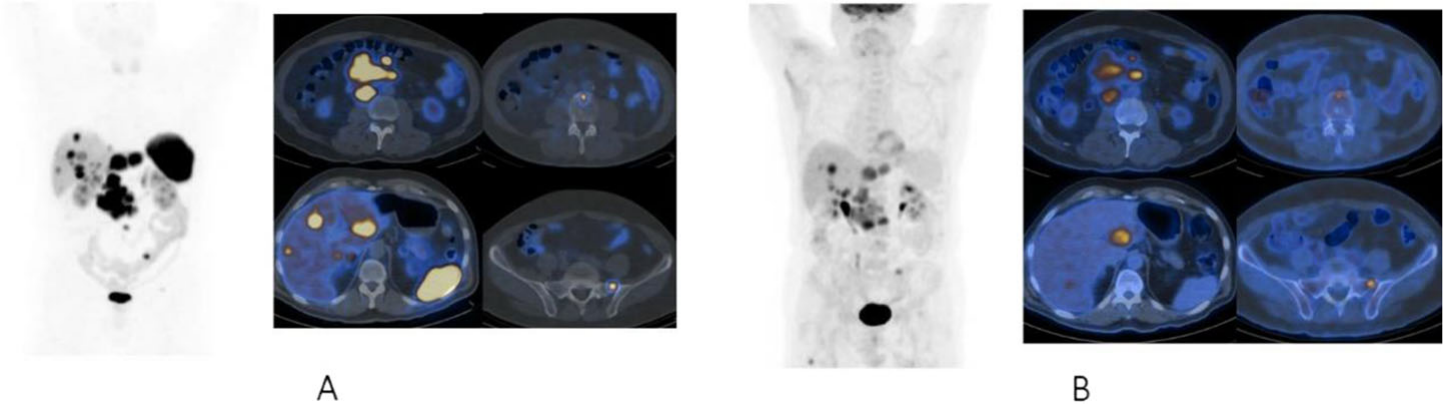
^68^Ga-DOTA peptide, staging NET of the pancreas, comparison with FDG. *Clinical history*: 68 y.o. man with moderately differentiated multi-metastatic NET of pancreas (Ki67 8%). *PET/CT findings*: ^68^Ga-DOTANOC PET/CT shows intense pathologic uptake of somatostatin receptor analogue by the known pancreatic tumour, as well as in lymph nodes, multiple liver lesions, and previously unknown bone lesions (a). FDG-PET/CT confirms pathologic uptake of the tracer in pancreas and lymph nodes and in some of the known liver and bone lesions (b)
